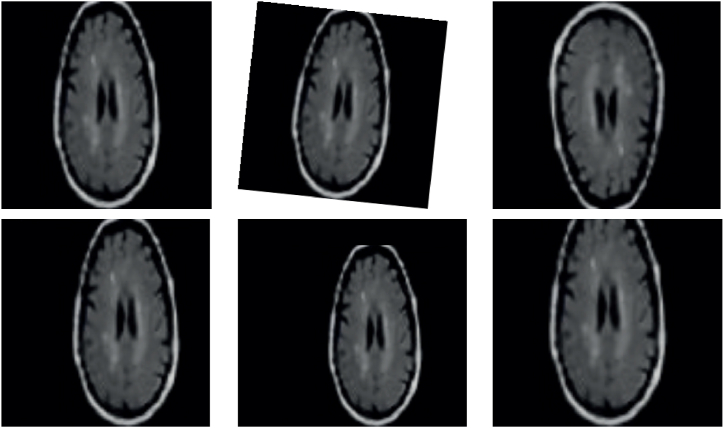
Collection of sample data augmented for the brain MRI.
